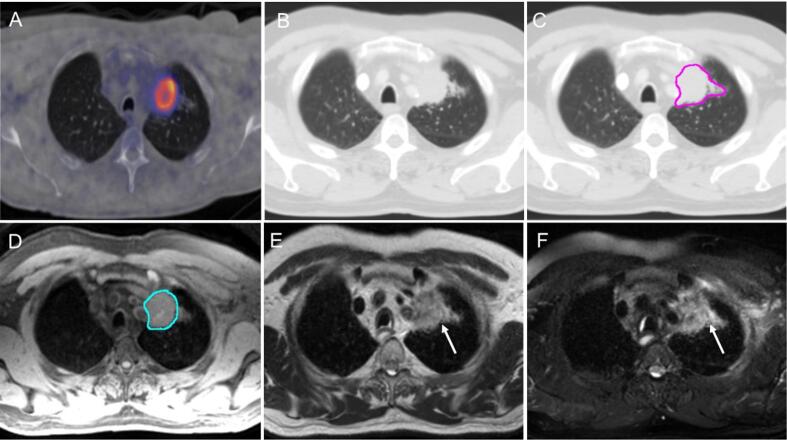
Parenchymal changes around the primary tumour; (A) PET-CT scan, (B) CT in lung windows (L: −474 HU, W: 1500 HU) without CT contour, (C) CT in lung windows with CT consensus contour (pink), (D) fat-suppressed T_1_w Radial GRE with MR consensus contour (light blue), (E) T_2_w TSE non-fat-sat demonstrating difference of signal intensity between tumour and atelectatic changes (arrow), (F) T_2_w TSE fat-sat demonstrating change of signal intensity (arrow). Signal intensity on the left side of the arrow was not included in the GTV as it was thought to represent adjacent atelectatic lung. (For interpretation of the references to colour in this figure legend, the reader is referred to the web version of this article.)
